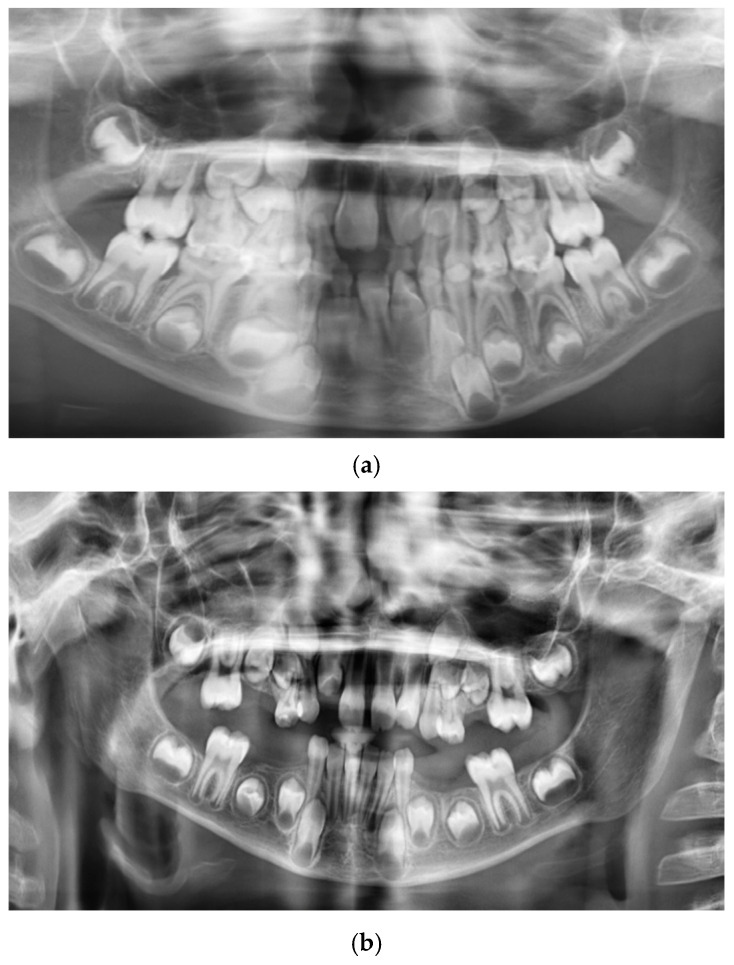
Patient n.2 pre- and post-treatment panoramic radiograph: (a) pre-treatment panoramic radiograph; (b) post-treatment panoramic radiograph.
